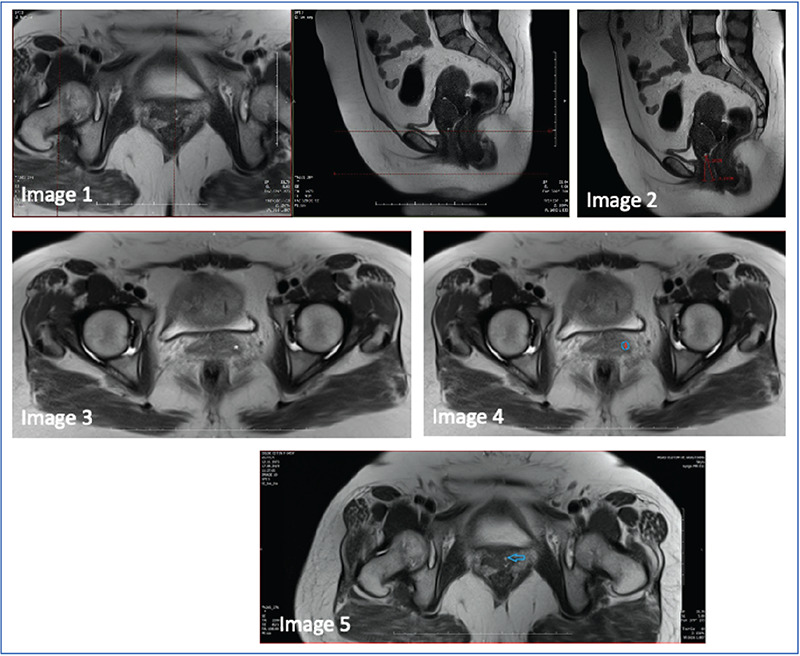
Image 1. Localization of hyperintense focus on axial and sagittal T2-weighted images of case number 13 Image 2. On sagittal T2-weighted image hyperintense focus and the distance between urethra-focus (first red line) and introitus-focus (second red line) of case 13 Image 3. Hyperintense focus on axial T2-weighted images marked with asterix the of case number 4 Image 4. Anteroposterior distance (red line) and area (blue line) of hyperintense focus on axial T2-weighted images of case number 4 Image 5. Hyperintense focus on axial T2-weighted image shown with blue arrow
